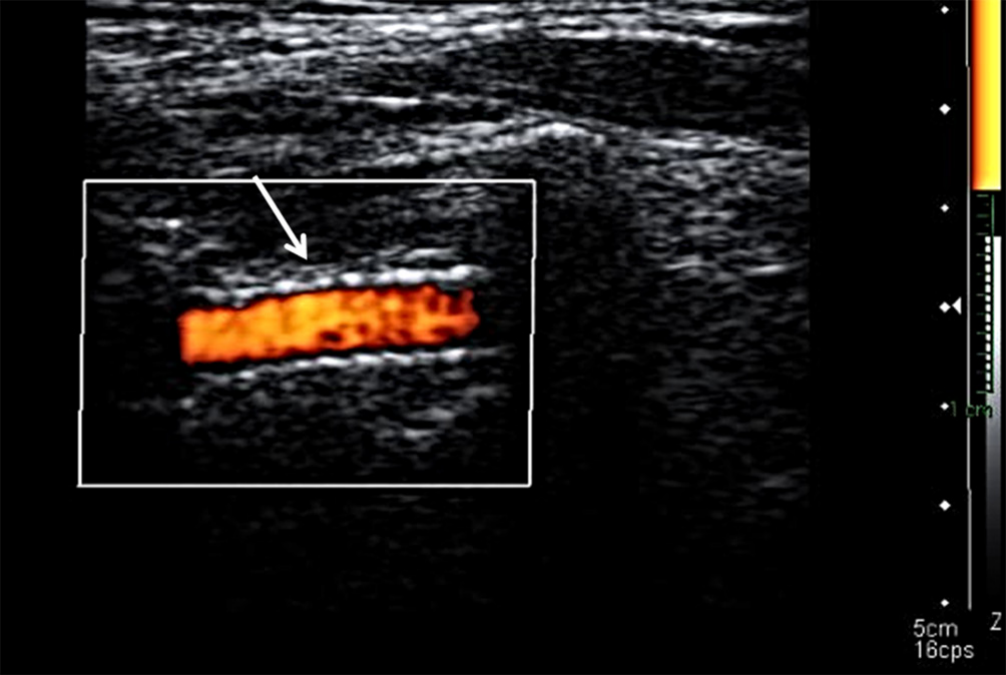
Left vertebral artery ultrasound. Doppler ultrasound performed during the 1-month follow-up visit. Ultrasound Doppler shows the implanted stent and normal flow inside.
